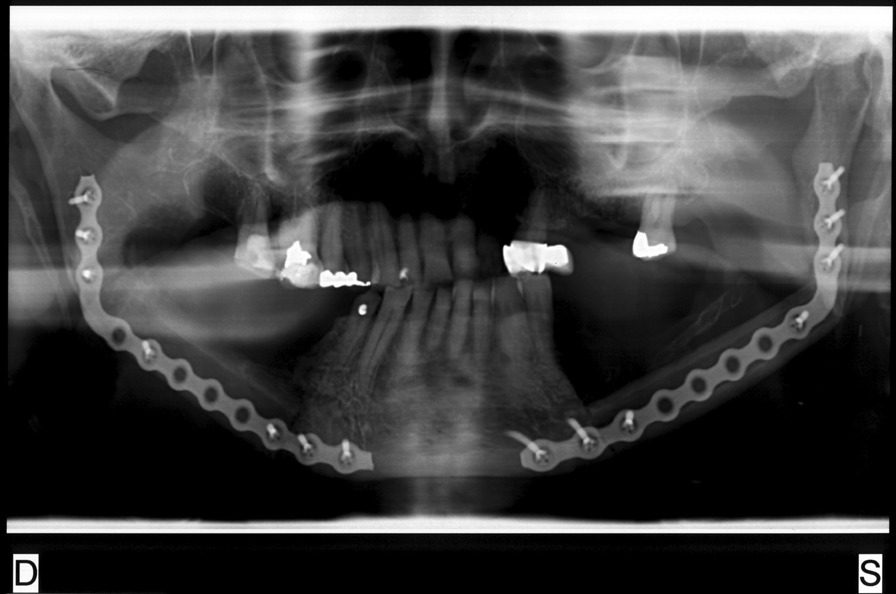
Postoperative orthopantomography
